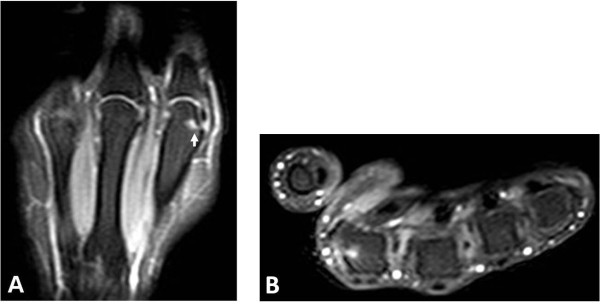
Fat-suppressed T1-weighted scans (A) coronal and (B) axial plane show an enhanced bone erosion (arrow). Rrepetition time/500 msec, echo time/16 msec. Enhanced bone erosion (arrow) in the head of the second metacarpal.
